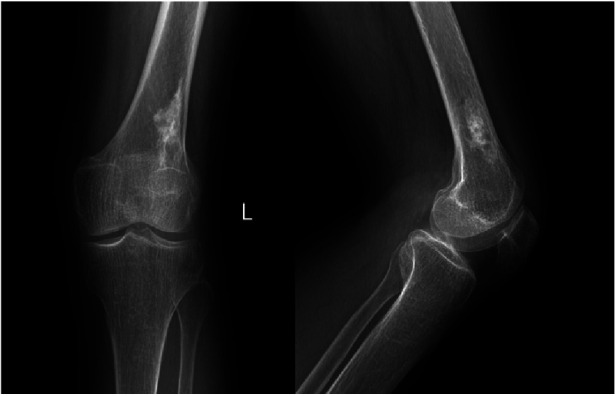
Anteroposterior and lateral radiographs of the left knee showing a patchy high-density shadow of the left distal femur without fracture or bone destruction.
